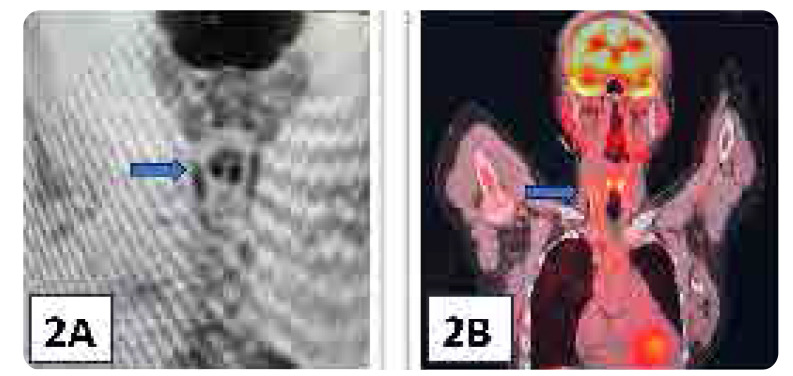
(A, B) Patchy FDG uptake in thickened walls of bilateral common carotid arteries with SUVmax (Maximum Standardised uptake value) of 7.2 (Blue arrows).
